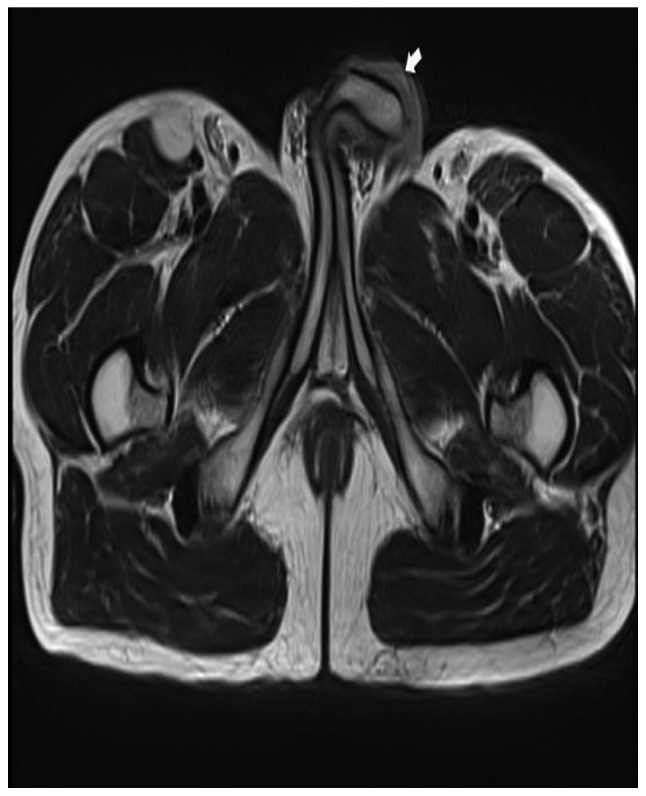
Magnetic resonance image of the pelvis. The white arrow indicates the abnormal lesion.
